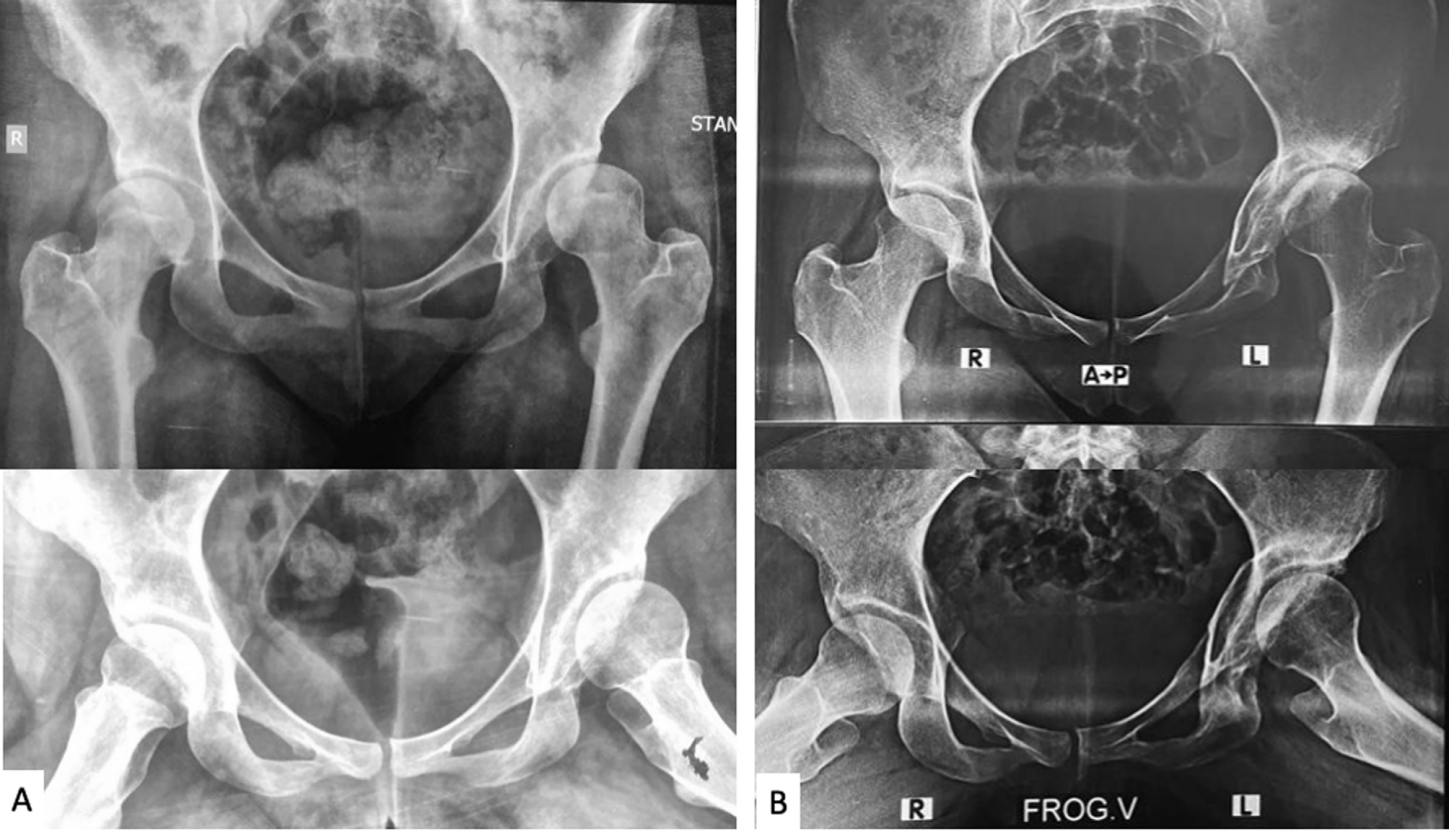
(A) Preoperative anteroposterior (AP) and frog-lateral radiographs of a 16-year-old female with left acetabular dysplasia. (B) 5-year postoperative radiograph demonstrating union of all osteotomies, maintained lateral acetabular coverage with a horizontal acetabular sourcil.
